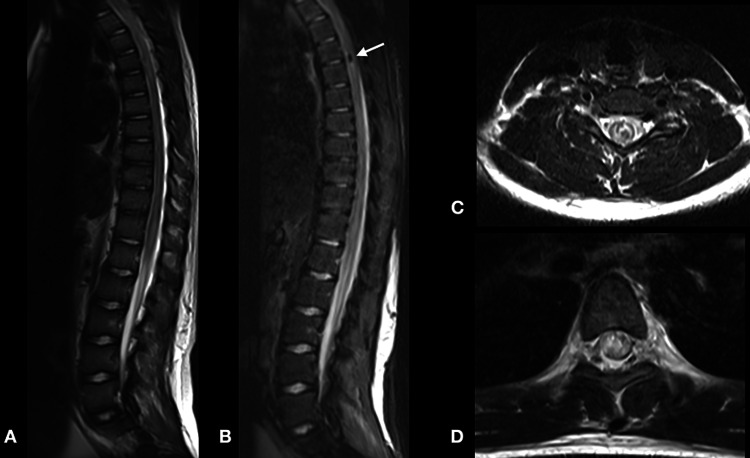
Sagittal T2-weighted MRI scans of the thoracic spine (A, B) and axial T2-weighted MRI scans (C, D) revealed the extensive spinal cord edema and signal changes suggestive of subacute hemorrhagic changes (white arrow).
